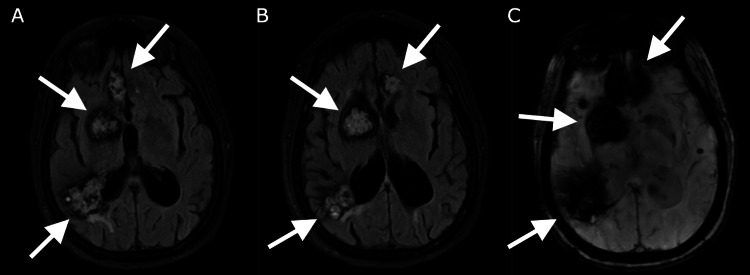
MRI brain with arrows indicating the patient’s known cerebral cavernous malformations as seen on (A and B) T2 FLAIR sequencing and (C) SWAN. FLAIR: fluid-attenuated inversion recovery; SWAN: susceptibility-weighted angiography
